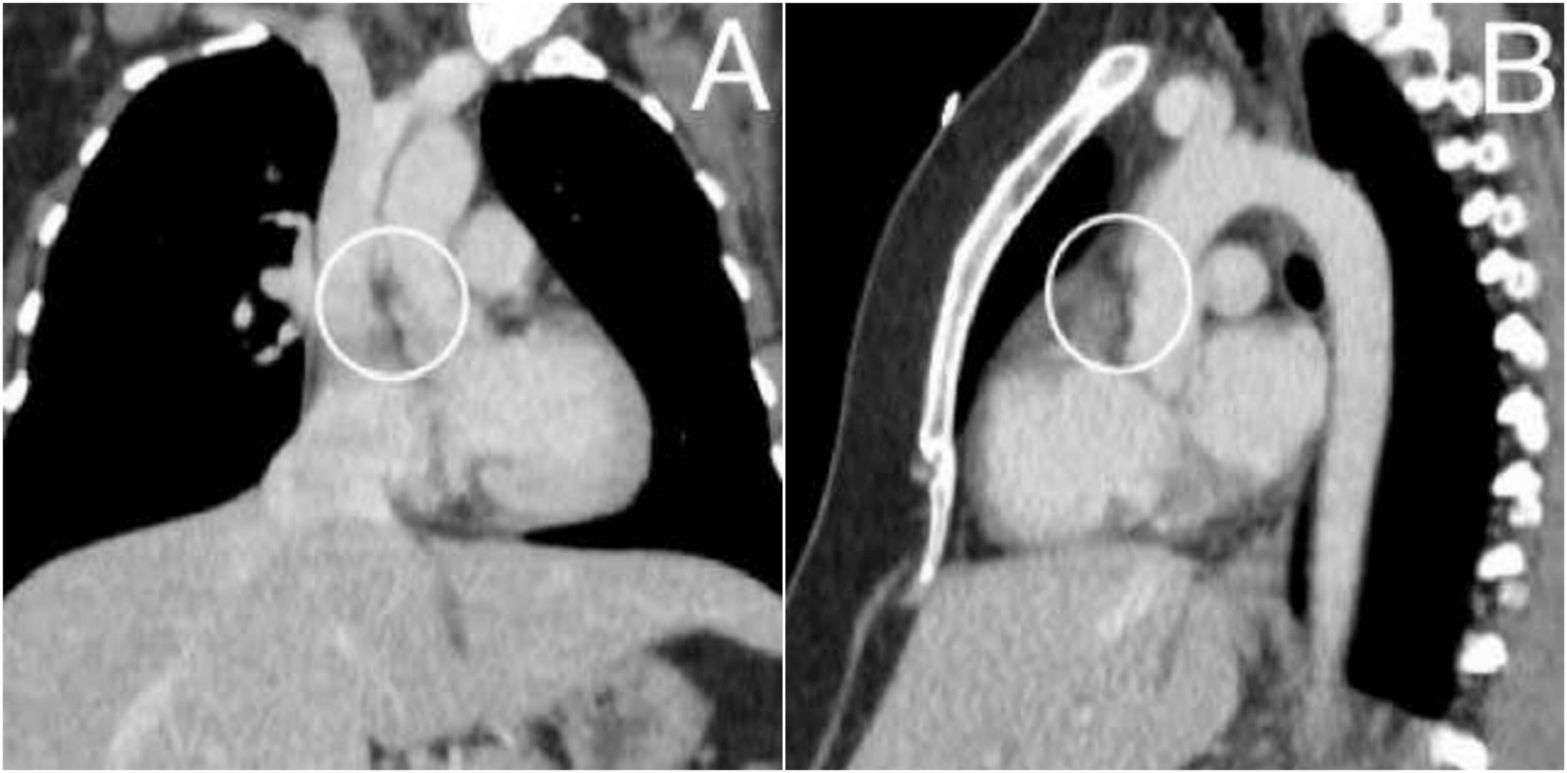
Thoracic Aortic Angio-CT coronal (A) and sagittal (B) slices showing no findings suggesting discontinuity of the intimal membrane at the aortic lumen level, nor filling defects or indirect findings suggesting pulmonary segmental branch thromboembolism, which translates into an absence of apparent vascular compromise. As an incidental finding, a hypodense, non-continuous line at the vascular lumen level is observed, not following typical trajectories, parallel to the acquisition plane, suggesting an artifact due to reconstruction or flow movement.
